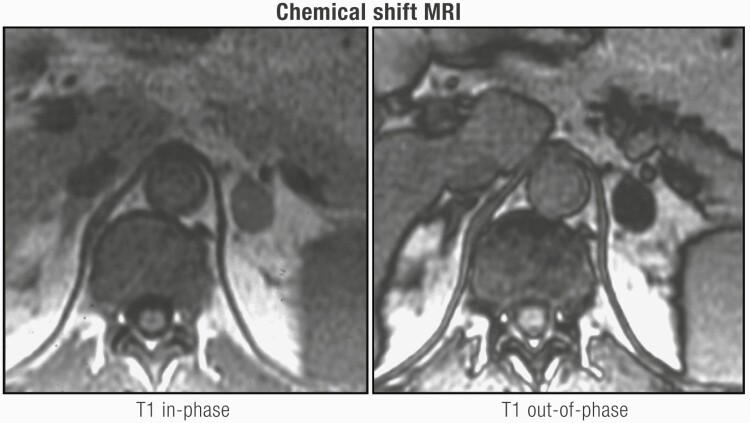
Chemical shift MRI. Paired axial T1-weighted MRI images showing loss of signal within a left adrenal nodule on out-of-phase imaging consistent with lipid-content within a lipid-rich adrenal adenoma.
